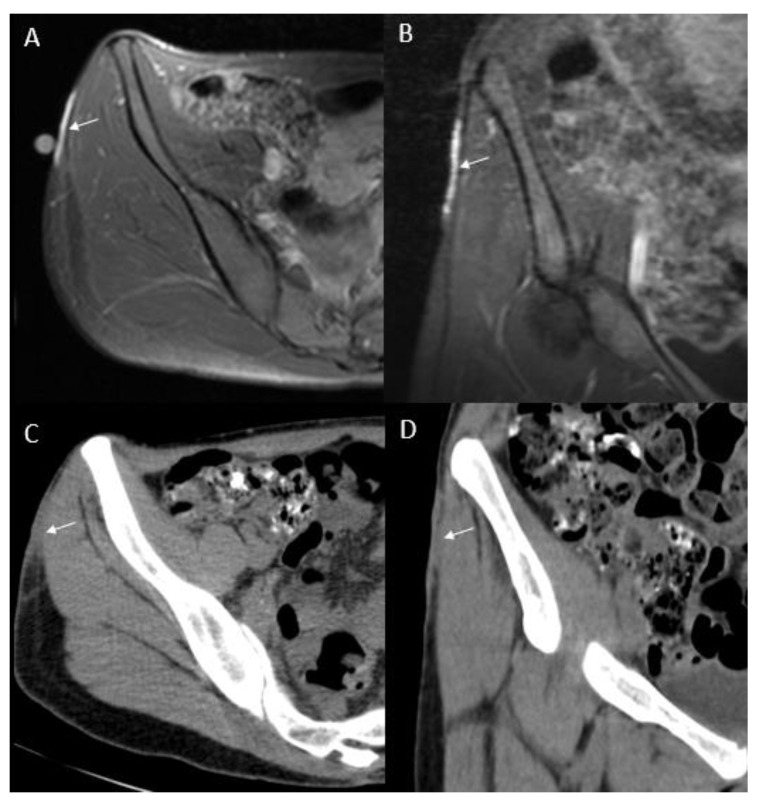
Magnetic resonance imaging (MRI) and computed tomography (CT) of a locally recurrent dermatofibrosarcoma protuberans (DFSP) of the right hip. (A) axial and (B) coronal MR T1-weighted fat suppressed post-contrast images of the right hip show enhancing tumor (arrows) diagnosed as a recurrent DFSP; (C) axial and (D) coronal corresponding images from non-contrast CT demonstrate soft tissue attenuation of the tumor (arrows).
